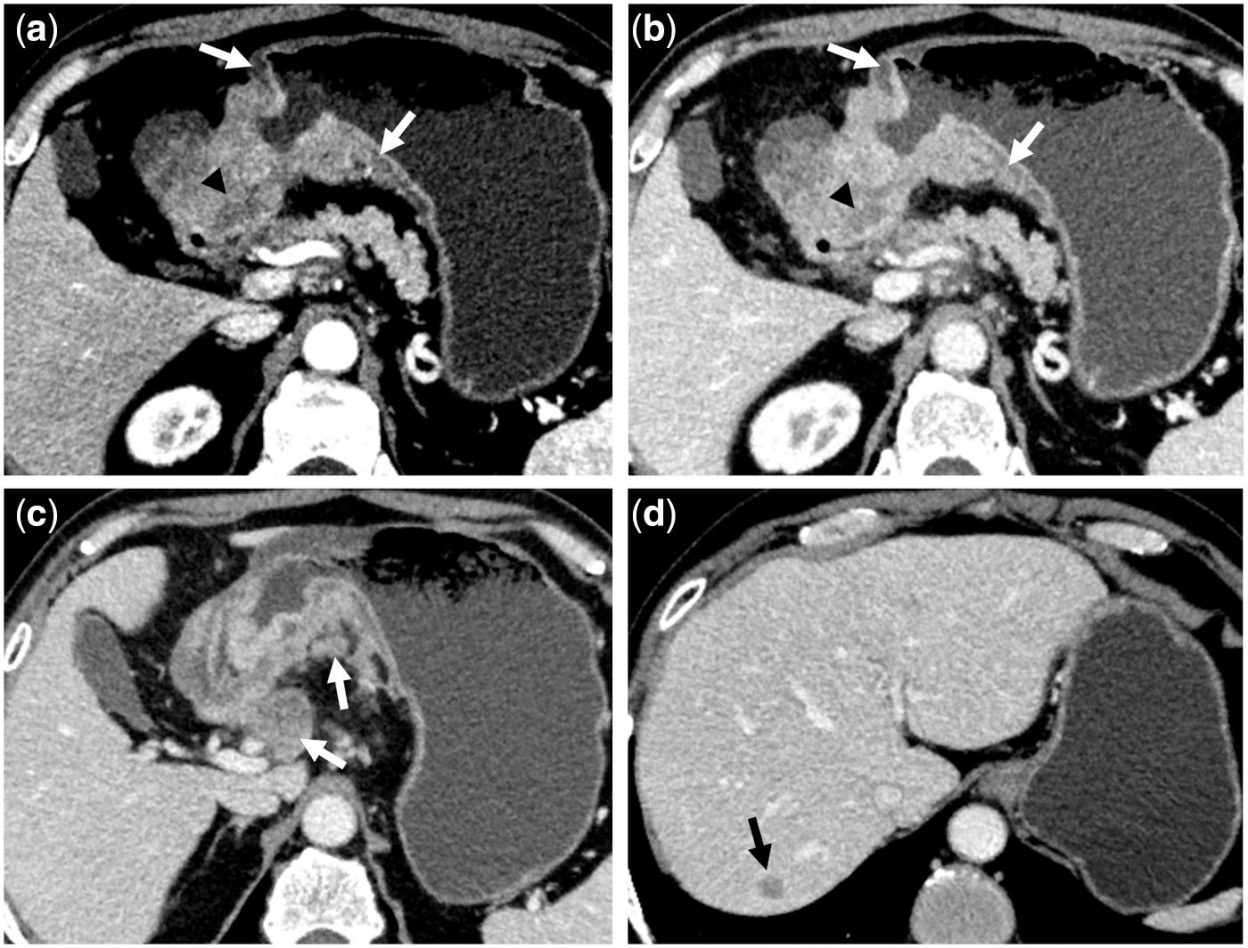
Multi-slice computed tomography scans of a 65-year-old male patient with human epidermal growth factor receptor 2-positive GC. Axial-enhanced arterial phase (A) and portal phase (B) computed tomography images show GC in the antrum and angulus of the stomach, with a poorly defined margin (white arrows in A and B), intratumoral necrosis (black arrowheads in A and B), enlarged perigastric lymph nodes with necrosis (white arrow in C), liver metastases (black arrow in D), and elevated serum cancer antigen 125 level. GC = gastric cancer.
